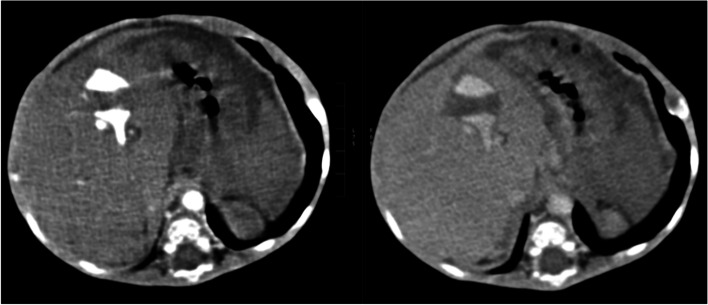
Axial CT arterial and portovenous images through the right lobe of the liver showing a tubular structure with early arterial filling and portovenous filling
